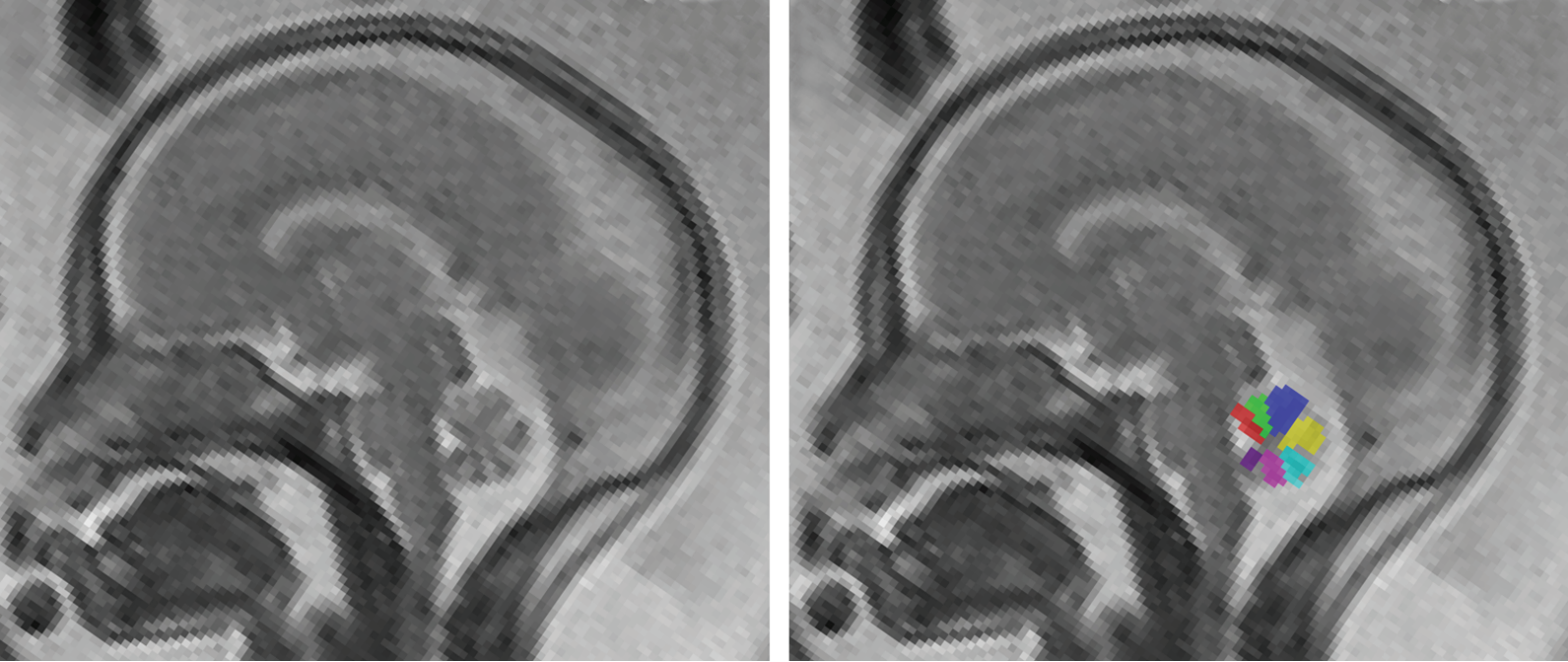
Midsagittal T2‐weighted magnetic resonance image of normal fetal brain at 22 + 0 weeks' gestation, showing seven (of nine anatomically possible) differentiable vermian lobules segmented (colored areas). In older fetuses, up to nine lobules can be differentiated.
